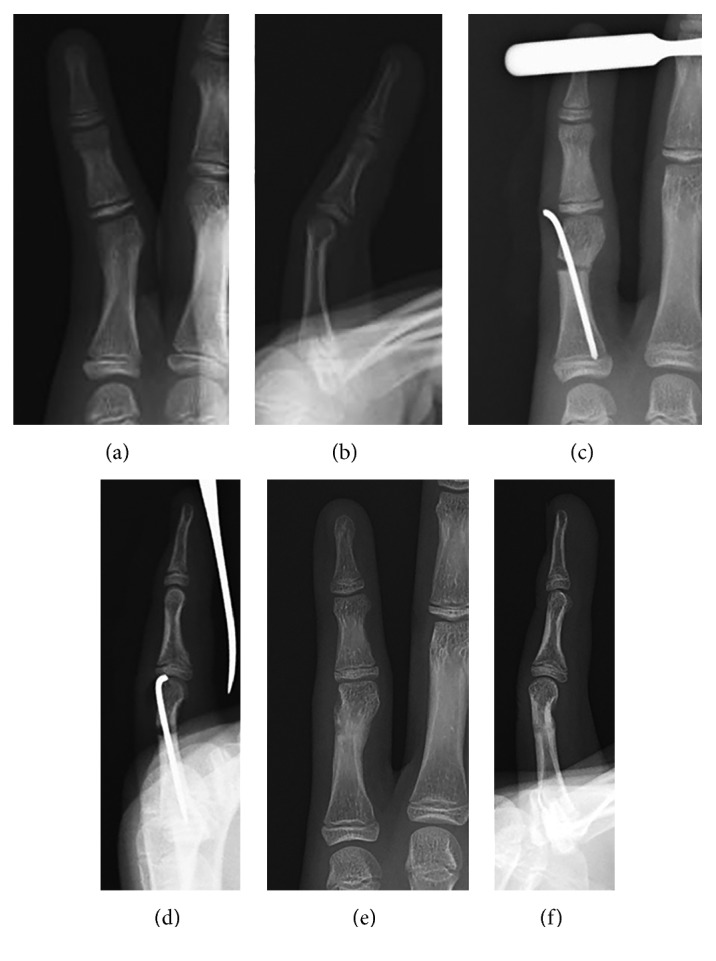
Radiographs of Case 2. (a, b) Preoperative radiography of the left little finger. (c, d) Images taken immediately after the surgery. (e, f) Images taken 3 months postoperatively.
